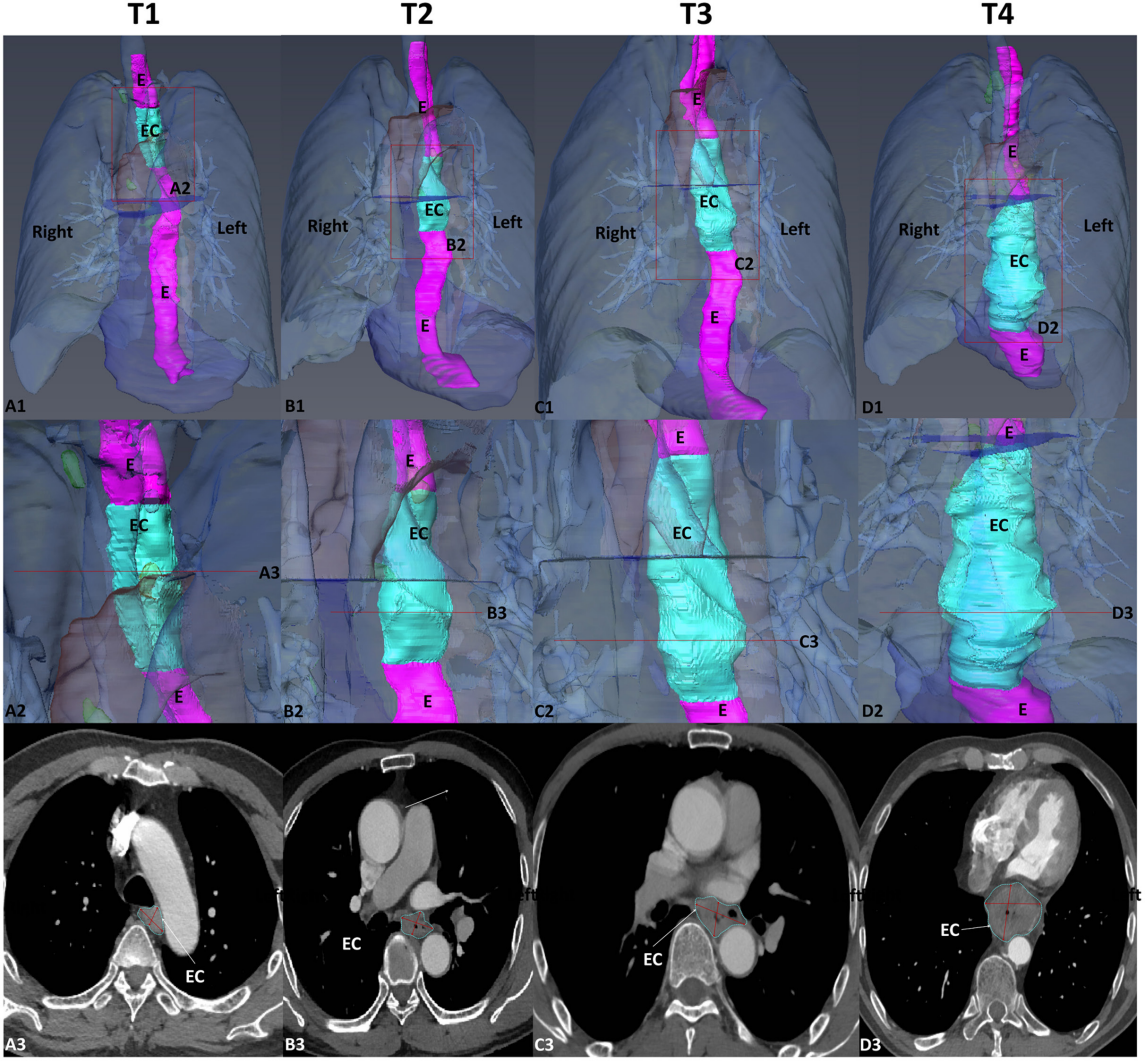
3D reconstructed model of EC. A1–D1:3D reconstruction of the four stages; A2–D2: local enlargements of the models; A3–D3: Transverse CTA images of the EC, including lines measuring the major and minor axis. E, normal oesophagus, EC, oesophageal cancer.
